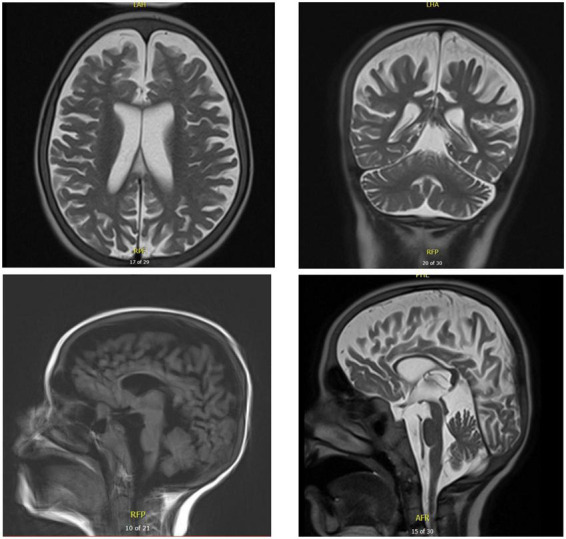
Brain MRI of patient five at the age of 17 years. T2 axial and coronal MRIs show general atrophy with normal white matter signal. Sagittal T1 and T2 brain MRIs show supra- and infratentorial cerebral atrophy, with marked thinning of the corpus callosum.
